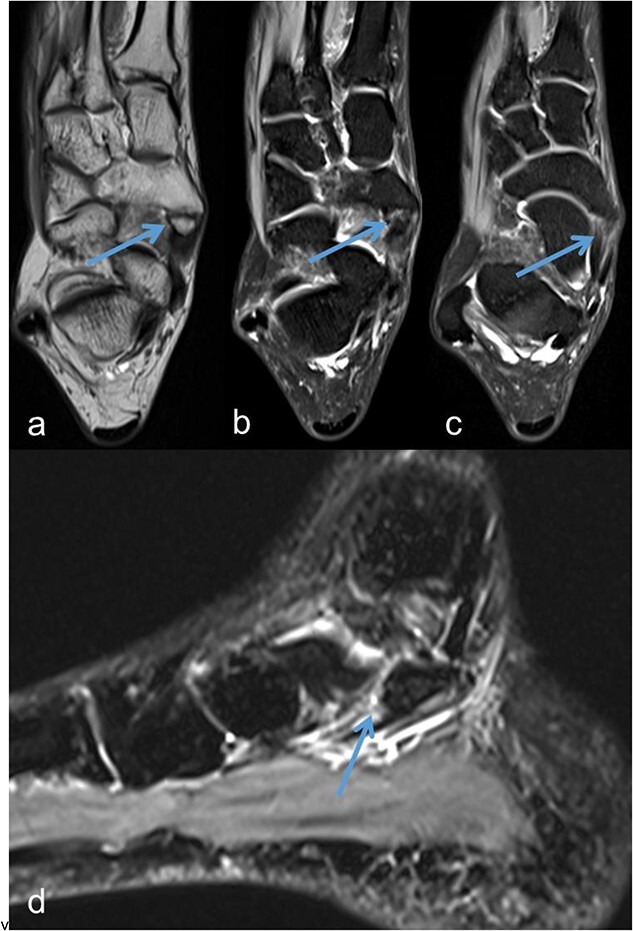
Os tibiale externum Type II in the coronal T1-weighted MRI scan (a), inflammatory reaction/tendinosis at the insertion of the tendon of the tibialis posterior muscle visualized in the heavily T2-weighted, fat-suppressed MRI scans (b and c), synchondrosis between the Os tibiale externum Type II and Os naviculare with central and perifocal edema visualized in the sagittal heavily T2-weighted, fat-suppressed MRI scan (d); each marked with an arrow.
